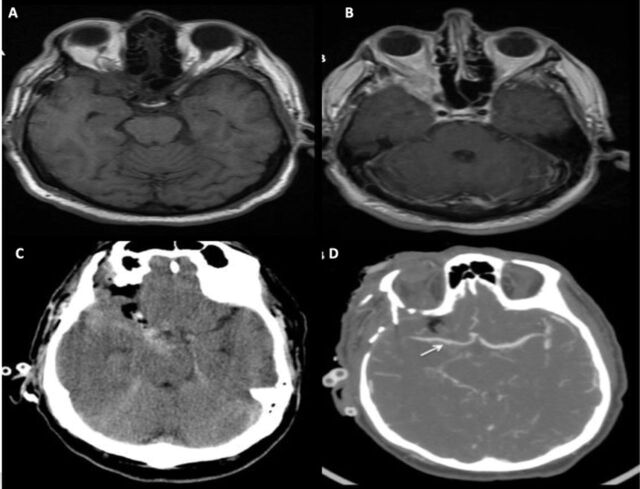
Axial magnetic resonance images of the brain (A) without and (B) with contrast show persistent enhancing right orbital apex mass prior to the planned ophthalmology procedure. (C) Axial computed tomography (CT) scan of the head demonstrates subarachnoid hemorrhage immediately following the transorbital approach to the orbital apex. (D) Axial CT angiography suggests mild irregularity of the right M1 segment (arrow).
